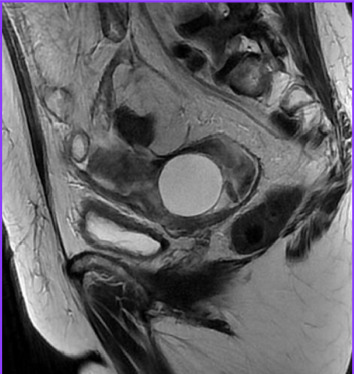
MRI T2 sequence, in a sagittal view, showing a complex tumor dependent on the left ovary.
